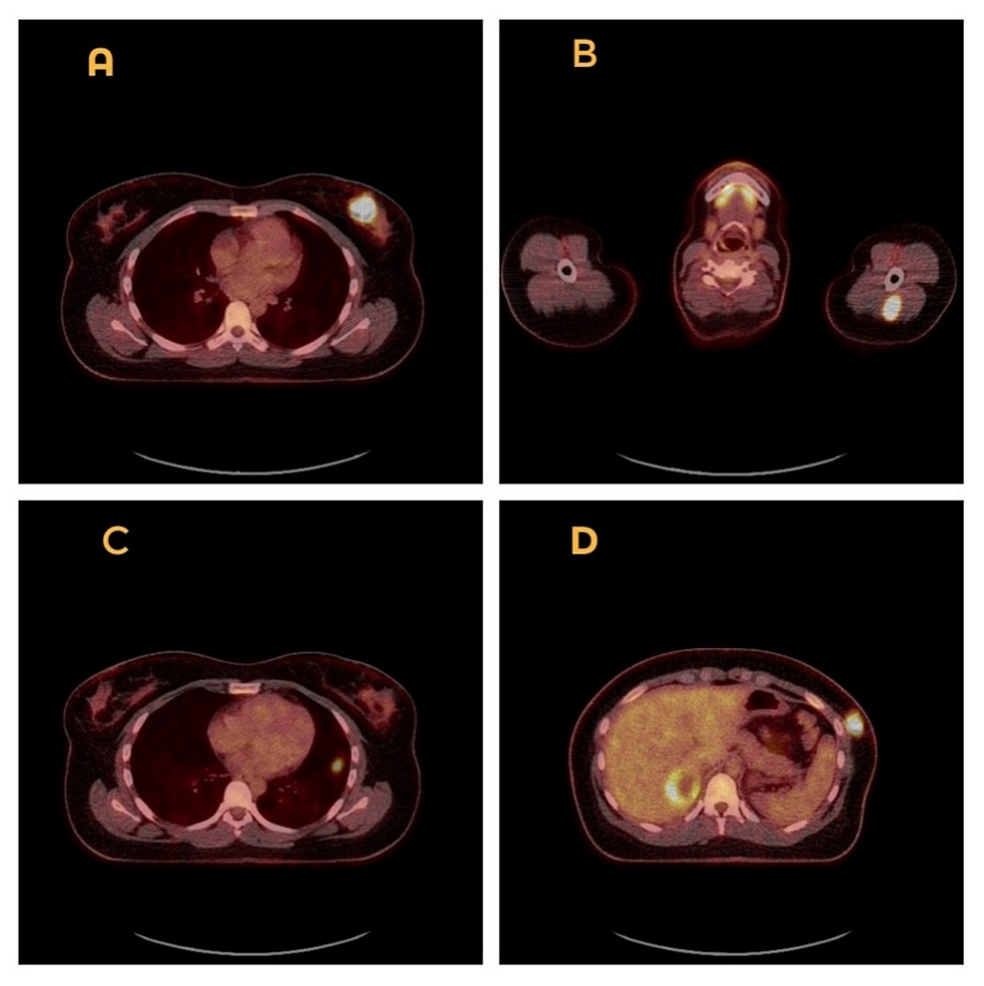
PET-CT scan showing fluorodeoxyglucose (FDG) avid lesions in the left breast (A) left upper arm intramuscular region (B), left lung (C), left lower chest wall (D).
